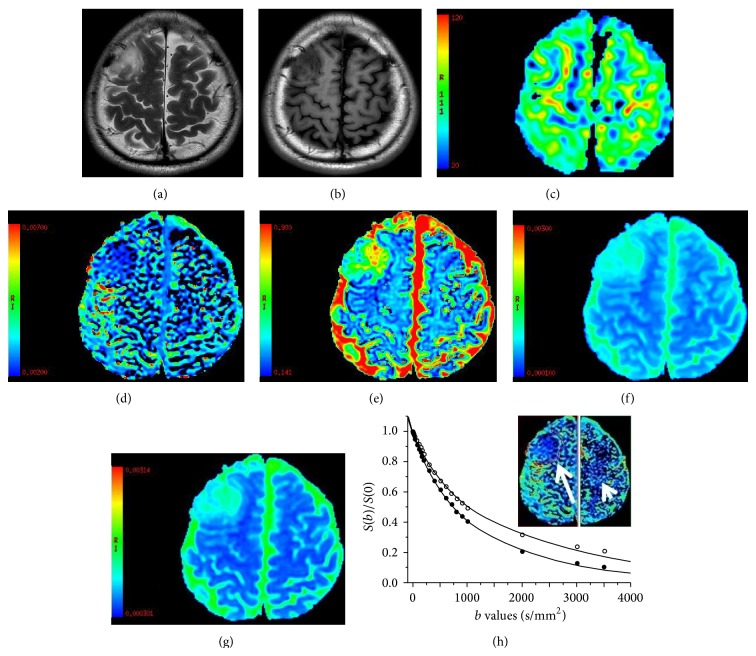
Male, aged 33 years with astrocytoma glioma in the right parietal (WHO II). The mass was hyperintensive with a fuzzy edge in axial T2-weighted image (a) and without any gadolinium enhancement (b). It shows slight perfusion in the CBF map (c) but fails to distinguish the boundaries of the tumor from normal brain substance. The TBF and CBF of contralateral semioval center were 80.72 and 27.53 mL/100 g/min, respectively. The *D*
